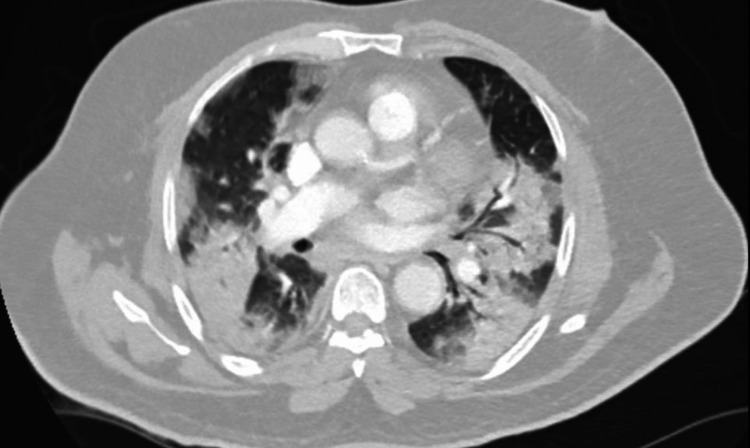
CT chest angiogram with contrast CT chest shows ground glass and consolidative opacities bilaterally with trace pleural fluid present bilaterally.
